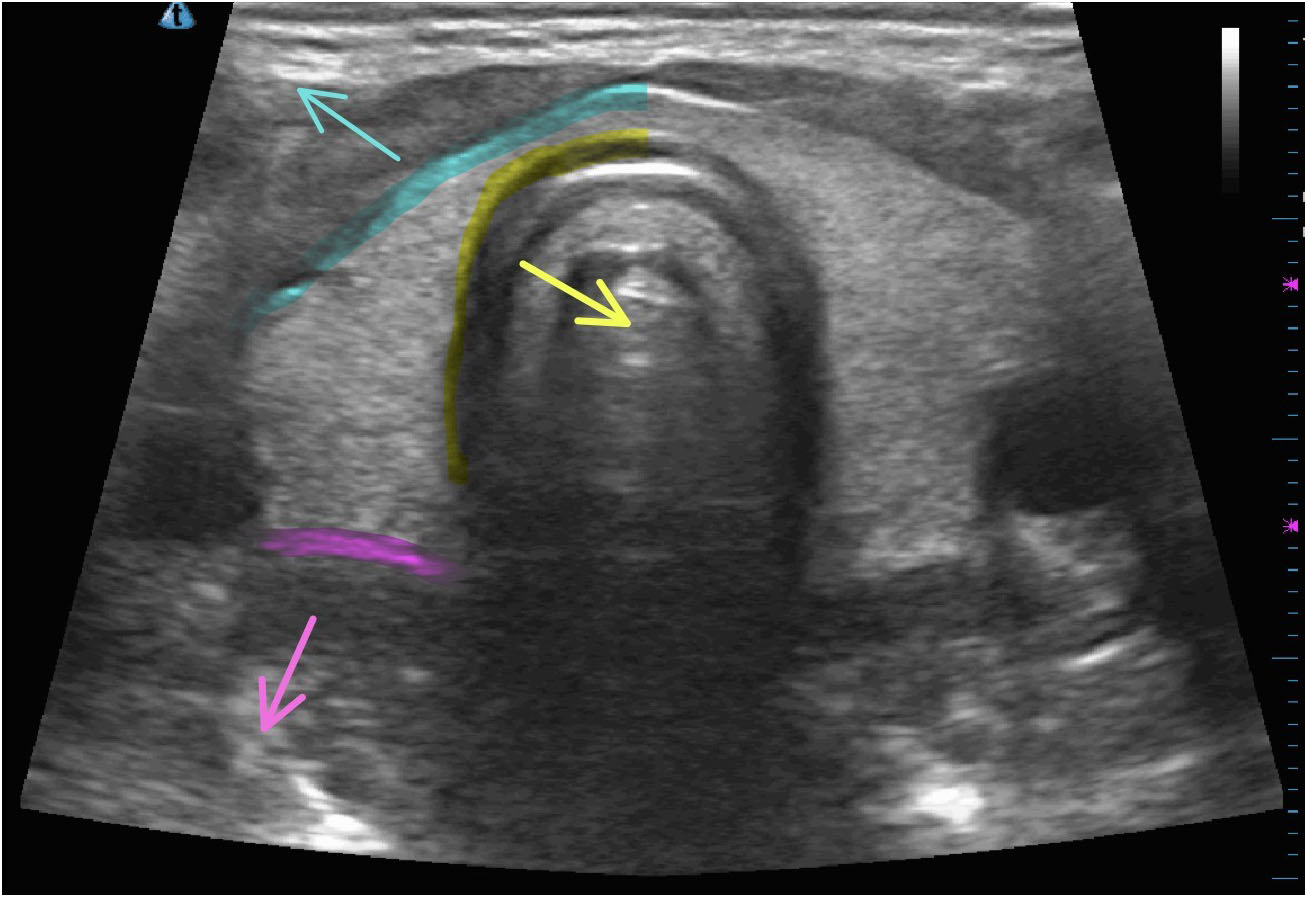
Locations of nodule rupture on normal thyroid ultrasonographic imaging with arrows indicating anterior rupture (blue), medial rupture (yellow), and posterolateral rupture (pink).
